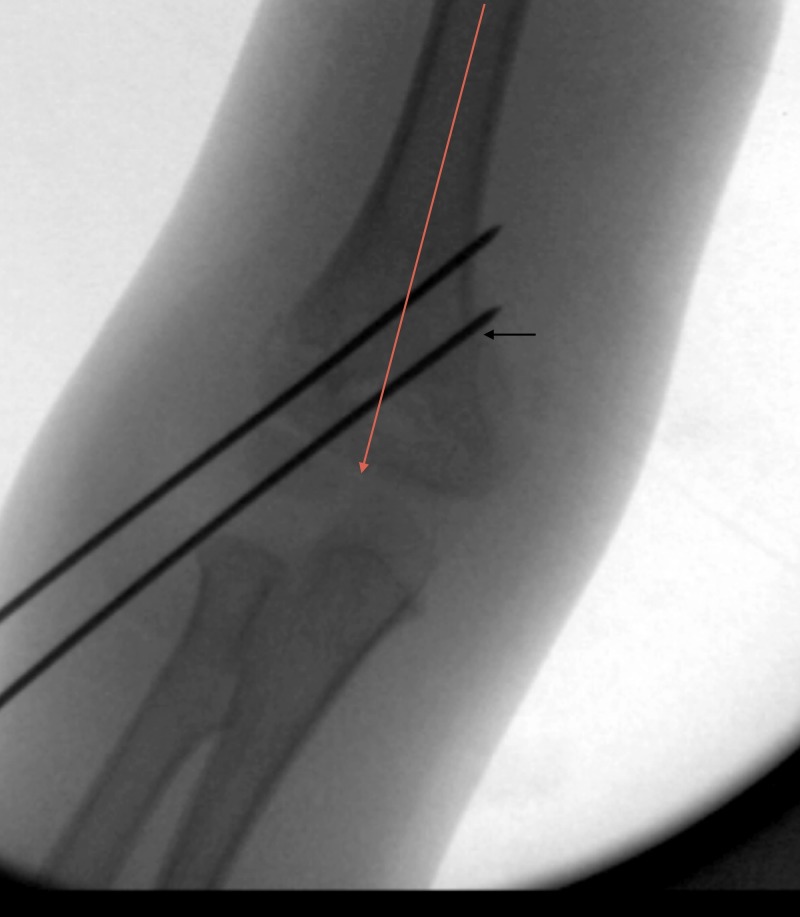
Intraoperative AP radiograph demonstrating fixation without bi-columnar fixation. The lateral wire (black arrow) does not have purchase in the lateral column distally. AP: anteroposterior
